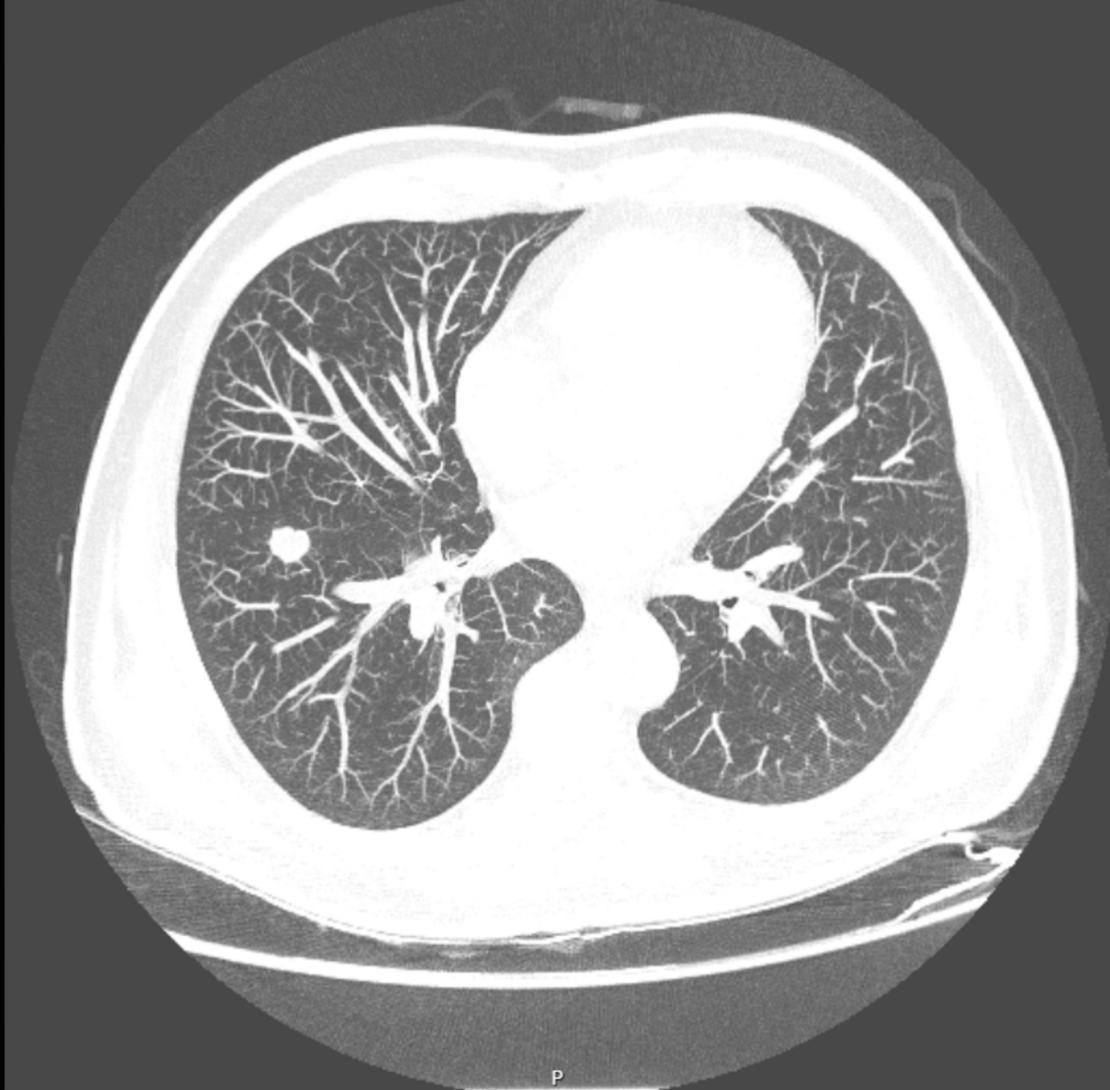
Chest CT (at initial diagnosis) Lung window, axial view. Initial presentation showing multiple pulmonary nodules consistent with metastatic disease. CT: computed tomography
